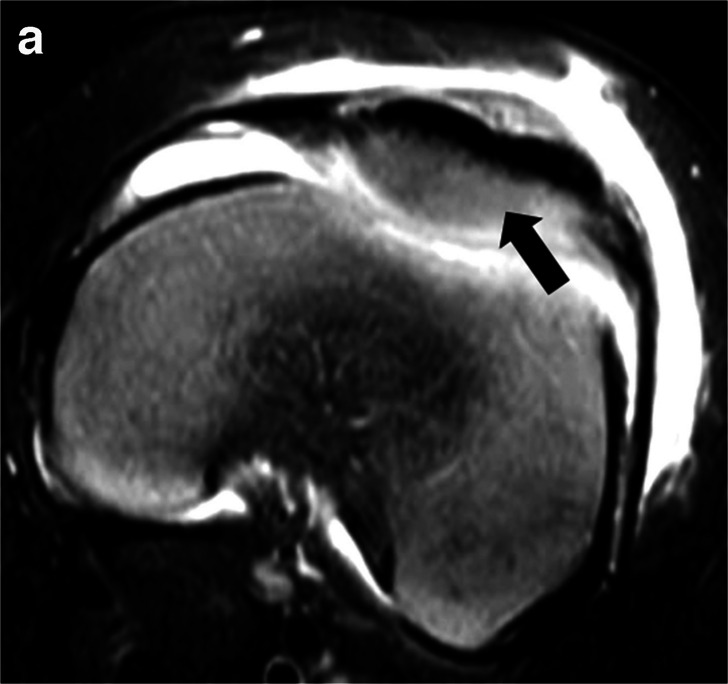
Unossified epiphyseal cartilage infection. A 14-month-old boy with septic arthritis and chondritis of unossified patellar cartilage. He presented to the ED with limp and fever. Axial T2W FS MR image (a) of the left knee shows subtle increased signal within the lateral aspect of the unossified patellar cartilage (*solid black arrow*). Axial T1W FS post-contrast image (b) shows lack of enhancement of this area (*solid black arrow*). The images additionally show a joint effusion with synovitis and inflammatory changes in the subcutaneous soft tissues
